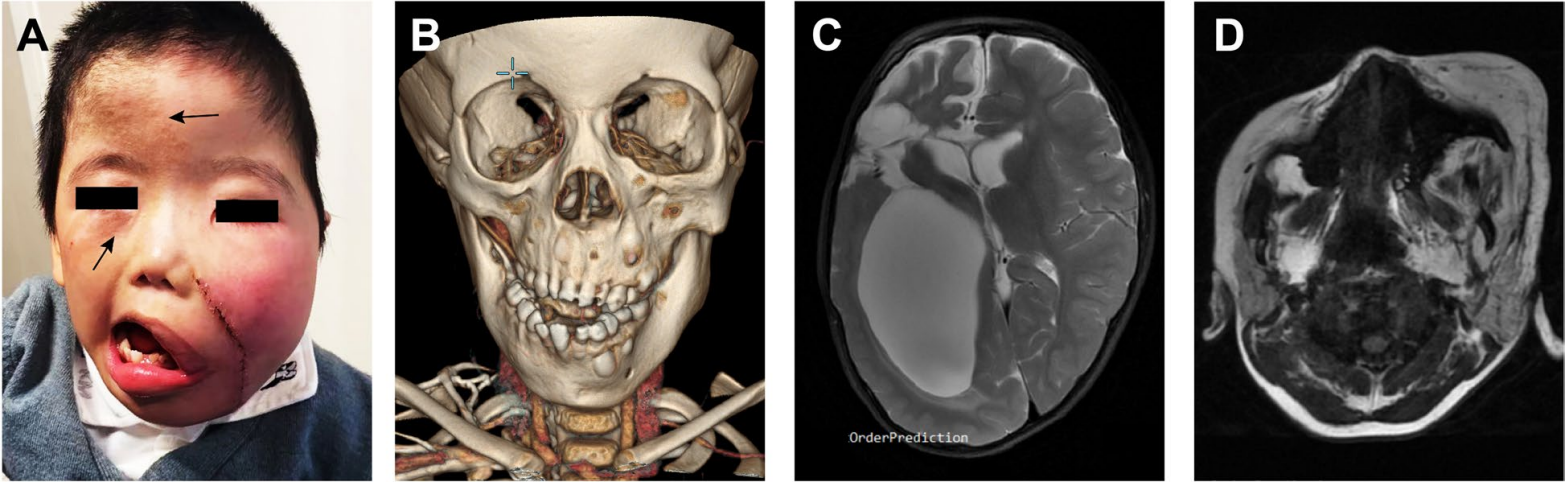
Phenotypic features of Patient 19. A Facial appearance was assessed. Epidermal nevi were observed in the right frontal and infraorbital regions. Despite undergoing debulking surgery at another center, facial asymmetry was still conspicuous. B BR revealed abnormalities in the zygomatic bone, maxilla, and mandible, along with dental misalignment. C T2-weighted axial image demonstrated hemimegalencephaly on the right side. The patient had previously undergone surgery for epilepsy. D T1-weighted axial image revealed extensive infiltration of fat into the soft tissues on the left side
